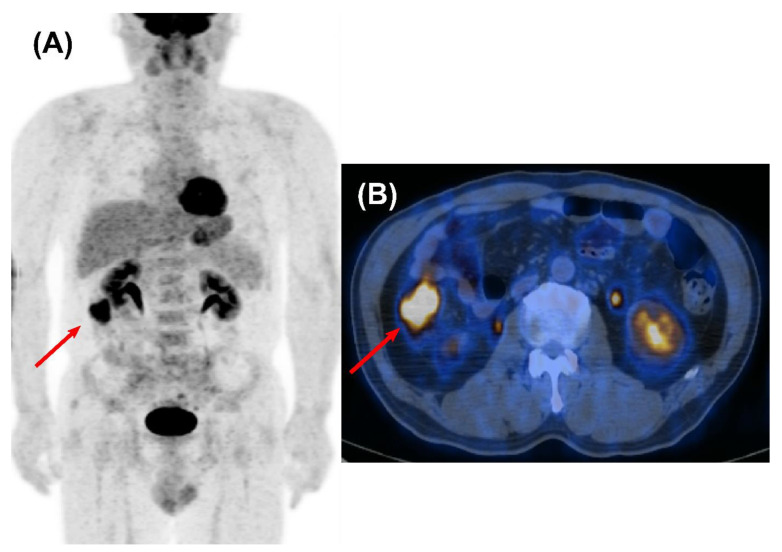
Second primary colon cancer detected by surveillance FDG PET/CT in a 52-year-old male who underwent curative surgery for NSCLC. A maximum intensity projection (MIP) (A) and a transaxial fused PET/CT image (B) obtained at 4 years after curative resection demonstrated a hypermetabolic lesion on the ascending colon without evidence of metastasis (red arrow). It was identified as primary colon cancer after surgical resection, and he has survived for more than 10 years after initial diagnosis of NSCLC.
